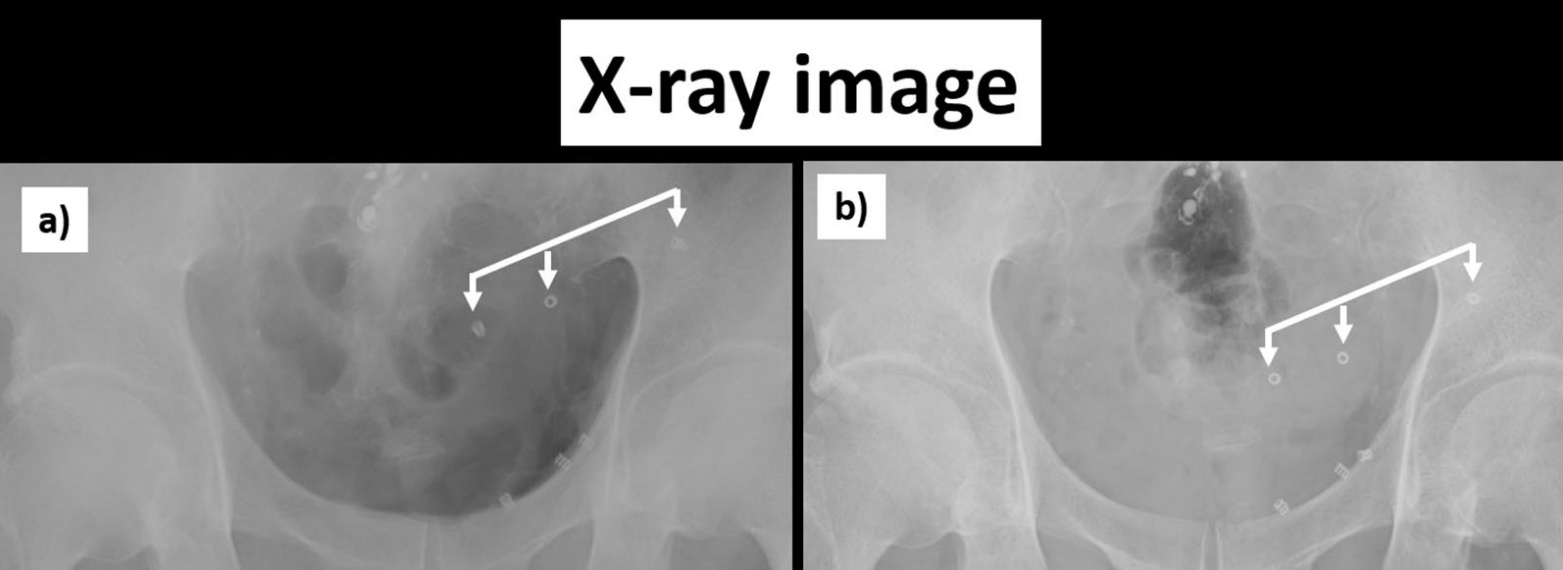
Abdominal x-rays after surgery (a) immediately after surgery; (b) 1 month after surgery) for the case with the greatest mesh shrinkage. The positions of 3 tackers placed on the ventral side (back surface of the rectus abdominal muscle, inner edge of the inferior epigastric artery and vein, and outer upper edge of the mesh) moved toward the dorsal aspect of the pubic bone and Cooper’s ligament, which were in the deepest part of the surgical field. The positional relationships of these 3 tackers were maintained as they moved.
